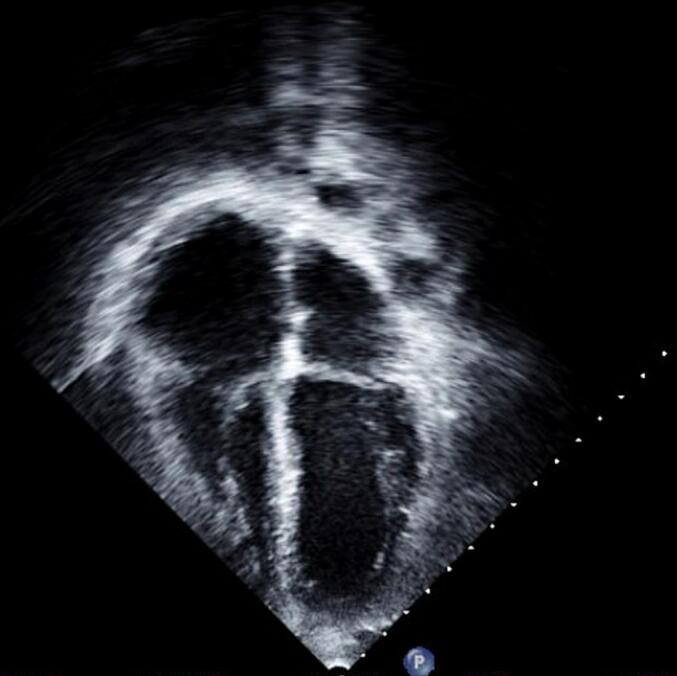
Transthoracic echocardiogram (TTE) obtained after repair of TAPVC and ASD. This figure shows absence of pericardial effusion on TTE obtained after repair of TAPVC.
